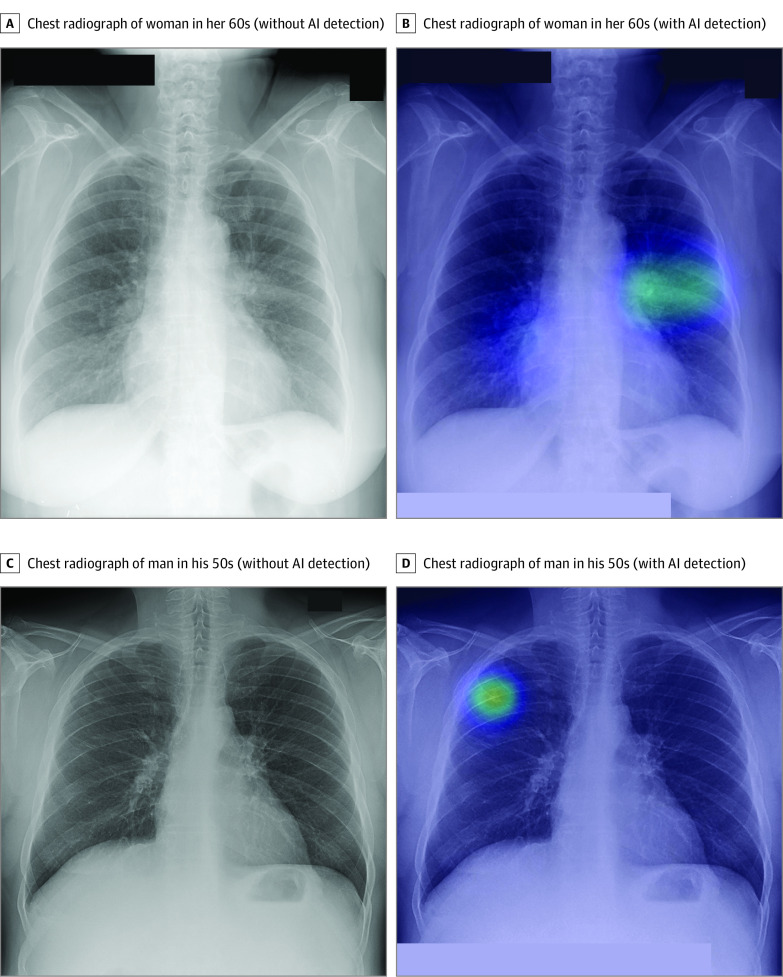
Frontal Chest Radiographs of Patients With Malignant Pulmonary Nodules Missed by NLST Radiologists But Detected by Artificial Intelligence Algorithm A, Chest radiograph of woman in her 60s (without AI detection). The woman was diagnosed with lung cancer 86 days after baseline imaging. B, Chest radiograph of woman in her 60s (with AI detection). The AI algorithm detected the missed subtle abnormality (in green, with nodule score of 38%) in the left perihilar region. C, Chest radiograph of man in his 50s (without AI detection). The man was diagnosed with lung cancer 127 days after baseline imaging. D, Chest radiograph of man in his 50s (with AI detection). The AI algorithm detected the missed subcentimeter nodule (in green, with nodule score of 53%) in the right upper lung zone. AI indicates artificial intelligence; and NLST, National Lung Screening Trial.
